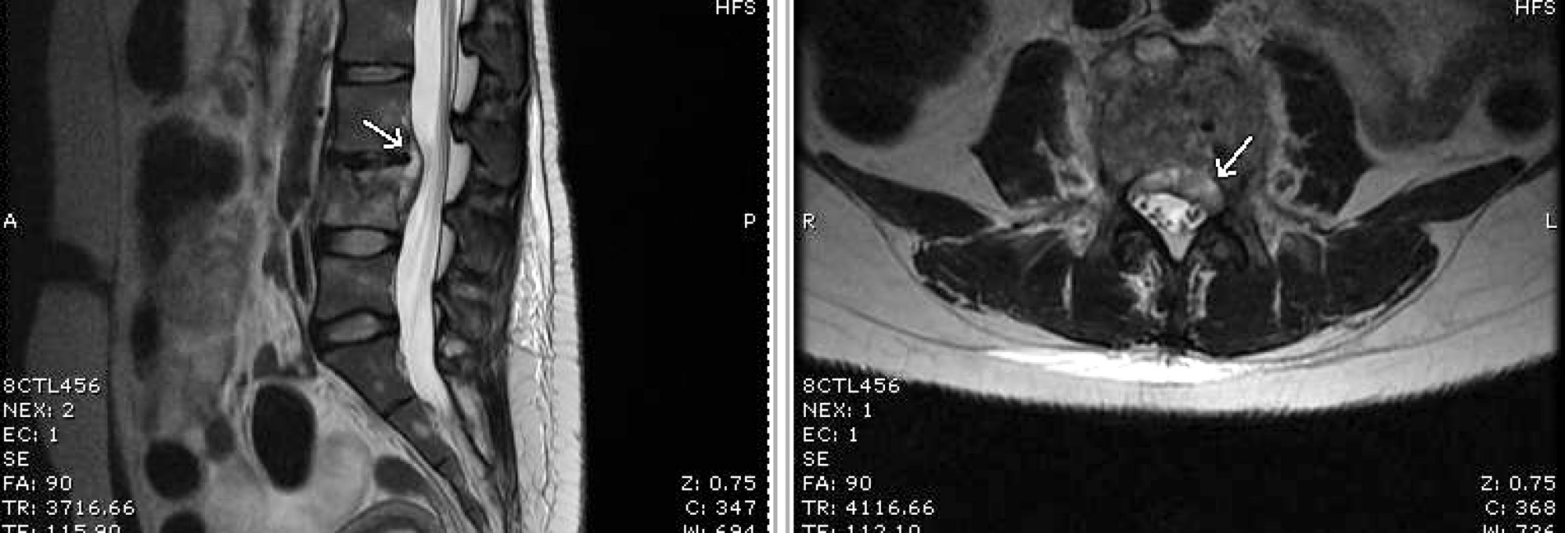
Follow-up lumbar MRI Magnetic resonance imaging (MRI) study with sagittal (left) and axial (right) T2-weighted views showing signal change within the L4 vertebral body with subligamentous spread (arrow on sagittal view) and an epidural component and sub-articular involvement (arrow on axial view)
